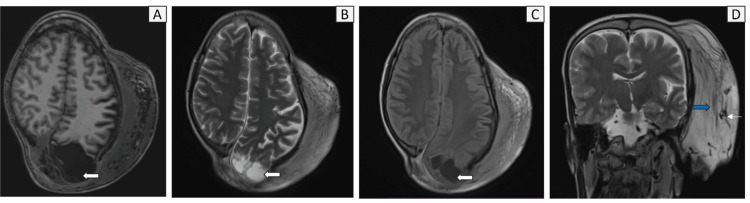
(A) Axial T1, (B) Axial T2, (C) Axial fluid-attenuated inversion recovery (FLAIR), (D) Coronal T2 shows T1 hypointense and T2/FLAIR hyperintense signals with multiple clustered linear fasciculo-nodular lesions (blue solid arrow) and prominent, serpiginous T2 hypointense flow voids (thin white arrow) representing vascular channels present within the lesion. There is a frank defect in the left occipital bone with a herniated meningocele (represented by solid white arrows).
